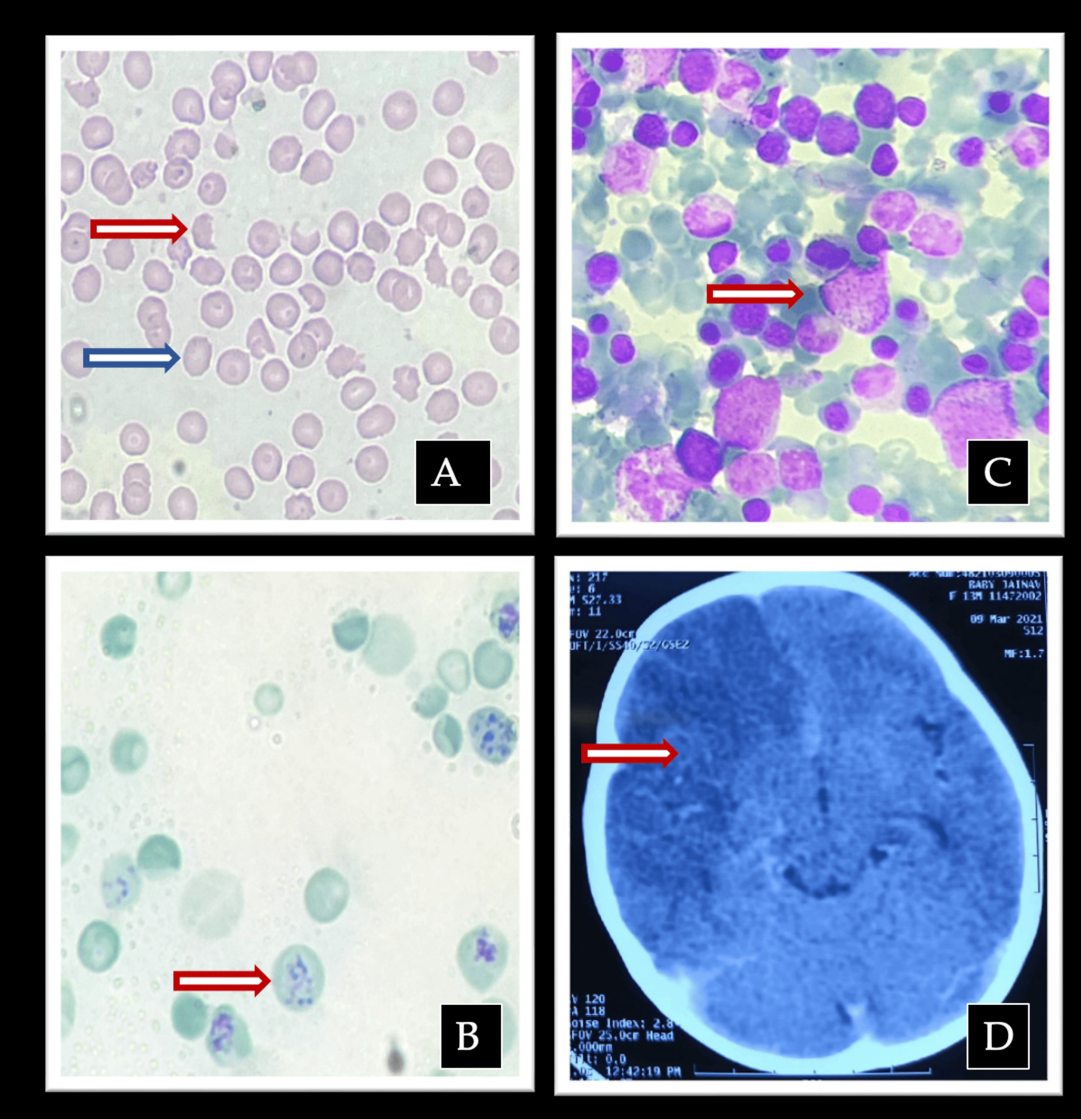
Hematopathological and neuroimaging findings of Case 2 2A: Peripheral blood smear showing schistocytes (fragmented red blood cells) and polychromasia, consistent with microangiopathic hemolytic anemia. The red arrow marks a fragmented red cell (schistocyte), and the blue arrow marks polychromasia. 2B: Reticulocyte preparation demonstrating increased reticulocytosis, reflecting marrow response to hemolysis. The arrow marks a reticulocyte. 2C: Bone marrow aspirate smear revealing reactive erythroid hyperplasia without blasts. An arrow marks an erythroid precursor cell. 2D: Computed tomography (CT) of the brain showing right middle cerebral artery infarction. The arrow marks a hypodense area in the right frontoparietal area suggestive of infarct.
